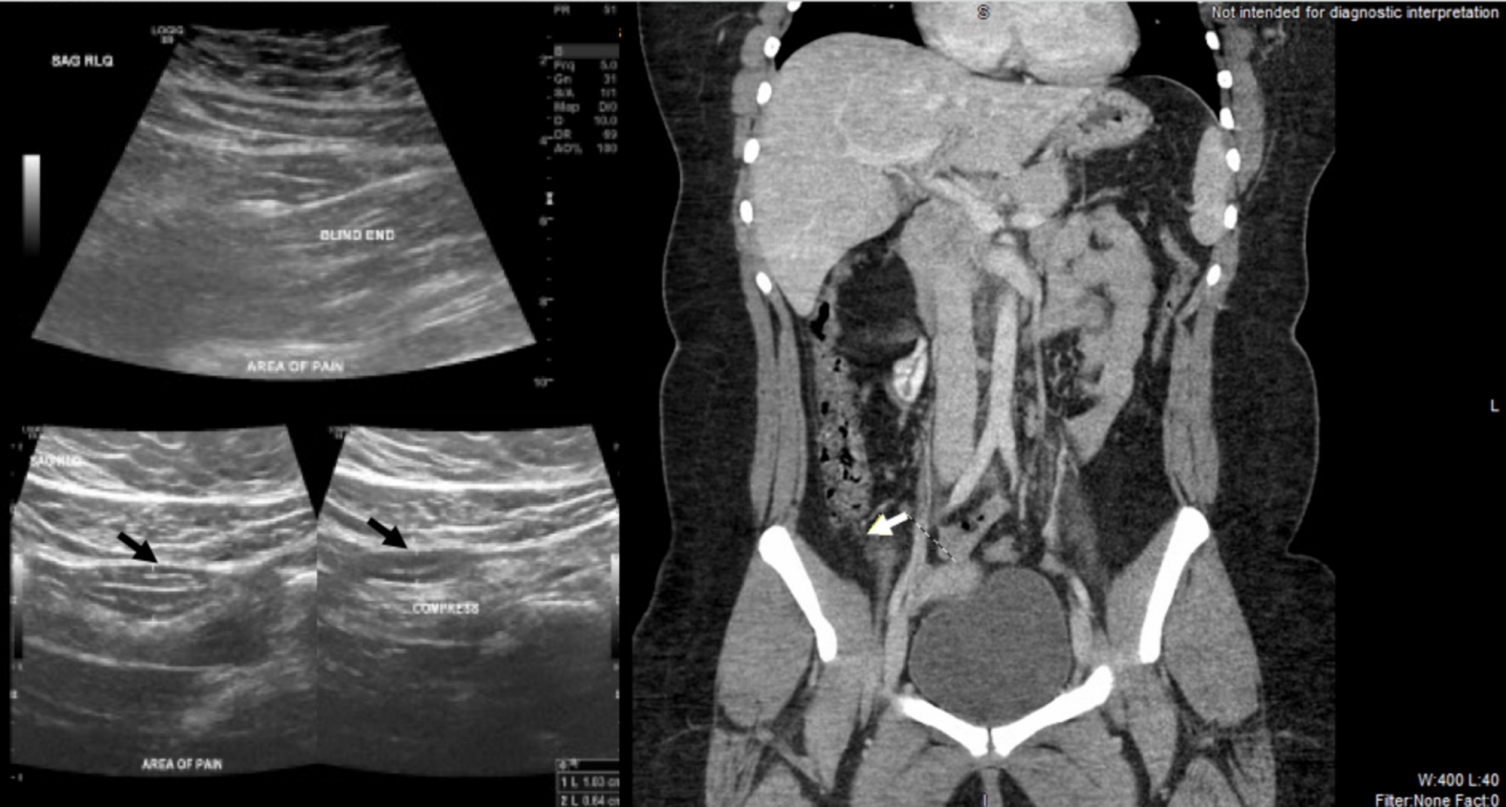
Ultrasound (left) shows non-compressible appendix (black arrow) and CT (right) shows normal appendix (white arrow)
